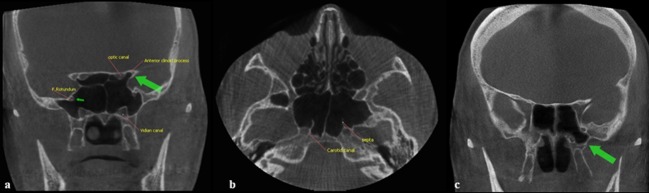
a: Coronal CBCT image: pneumatization of the bilateral anterior clinoid processes and protrusion of optic canal (note that the protrusion of vidian canal and foramen rotundum is bilateral), b: Axial CBCT image: pneumatization of the carotid canal and the septa inserting into its bony covering,  c: Coronal CBCT scan showing the pneumatization of the left pterygoid process (arrow) of the sphenoid sinus
